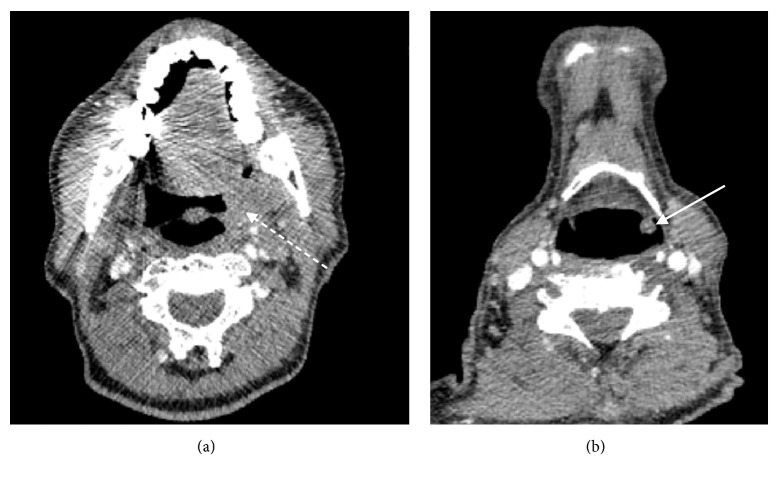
(a) Axial CT image with contrast at the level of the suprahyoid neck revealing an enlarged left palatine tonsil (dashed white arrow). (b) Axial CT image with contrast at the level of the hyoid bone which demonstrates a subcentimetre mucosal soft tissue nodule in the left aryepiglottic fold (white arrow).
